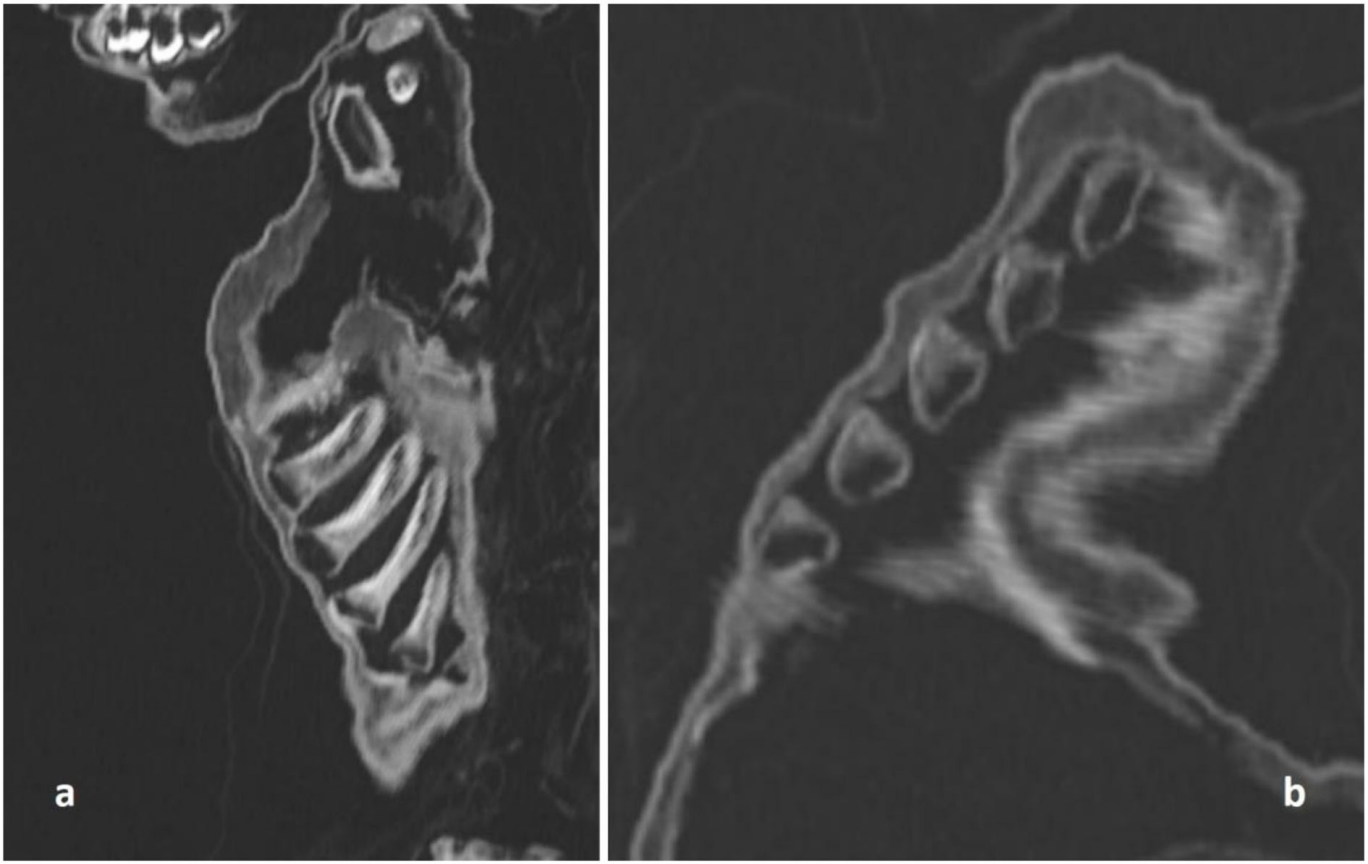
CT scan of the costochondral junction with an enlargement such as seen in “rickety rosary” but also in “scurvy rosary.” (a) Sagittal section; (b) oblique section.
